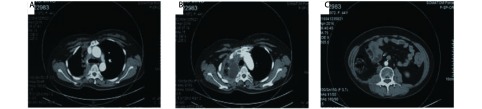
患者2013年术后影像检查。A：胸部CT提示：经手术后患者纵隔内肿物完整切除；B：胸部CT提示：经手术后患者纵隔内肿物完整切除；C：腰大肌旁肿物切除后肿物消失。 CT scan after the surgery in 2013. A: Chest CT indicates mediastinal tumor was completely resected. B: Chest CT indicates mediastinal tumor was completely resected. C: CT scan indicates tumor adjacent to the psoas major muscle was completely resected.
